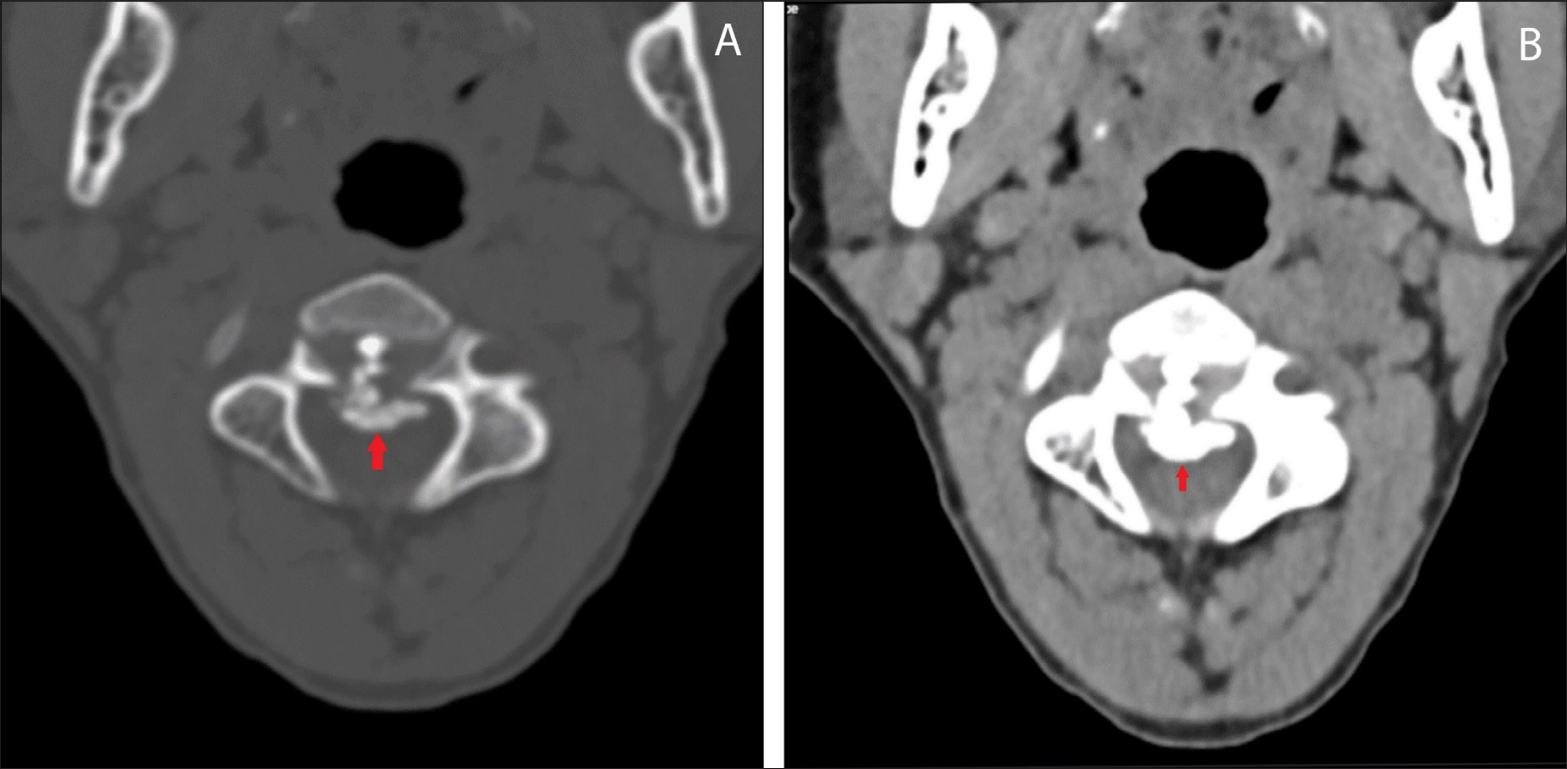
Subsequent magnetic resonance imaging (MRI) showed peripheral epidural enhancement surrounding the calcified disc with a tail-like extension superiorly and inferiorly (Figure 3A–B, sagittal and axial T1-weighted images [WI] after gadolinium contrast respectively, red arrow: herniation, asterisks: enhancement). Spinal cord compression is best appreciated on the axial image. Sagittal T2-WI revealed low signal intensity of the calcified disc herniation (Figure 3C, red arrow). The patient was treated conservatively with analgesics and a cervical collar. There was a significant improvement with gradual resolution of pain. Follow-up MRI four months later demonstrated nearly complete resolution of the extruded calcified disc.
